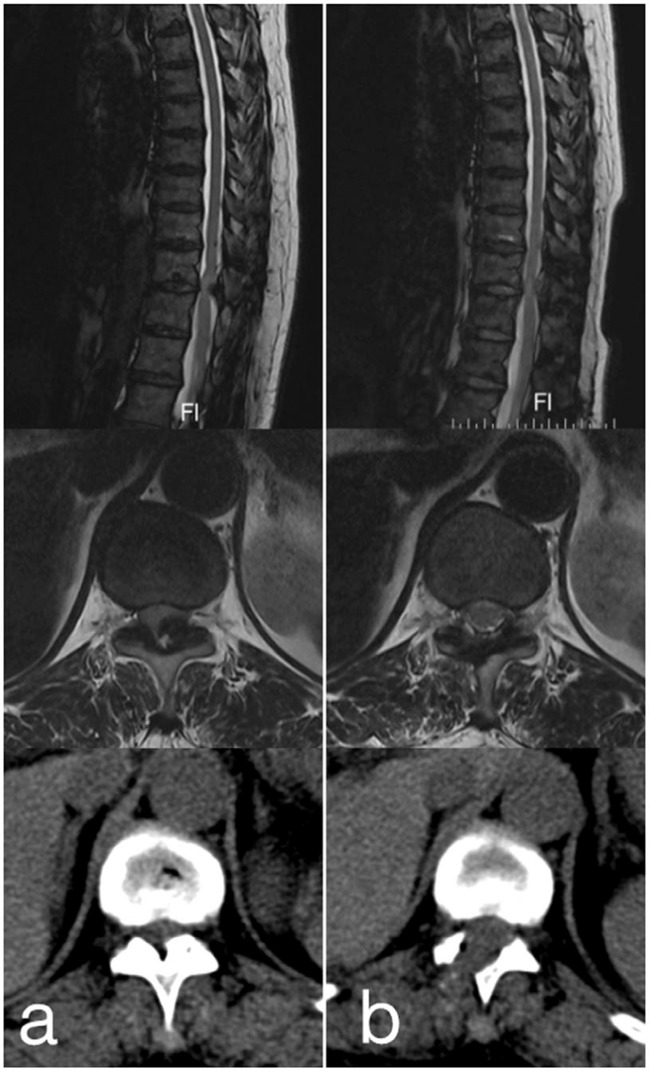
Pre- and postoperative images of PEPD (case 21). (a) Sagittal MRI, axial MRI and CT revealed the OLF at T11/12 and the compressed spinal cord. (b) Satisfactory decompression was completed with a dome-shaped laminotomy through limited laminectomy and flavectomy.
